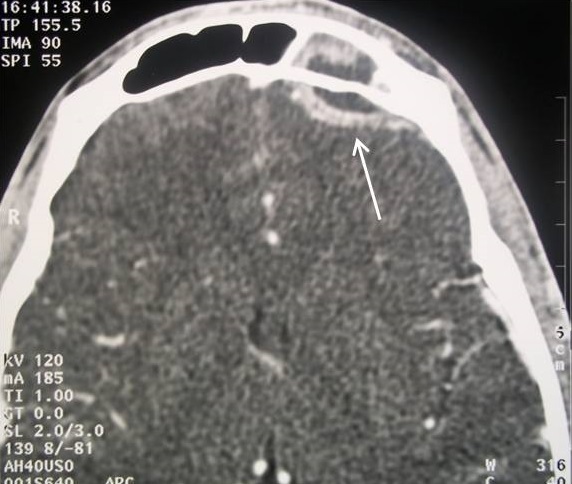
CT in axial cuts after PDC injection in parenchymatous window showing a hypodensic collection on both sides of the internal table of the left frontal sinus with peripheral contrast (arrow) in favor of a left frontal extradural empyema complicating sinusitis Frontal homolateral
